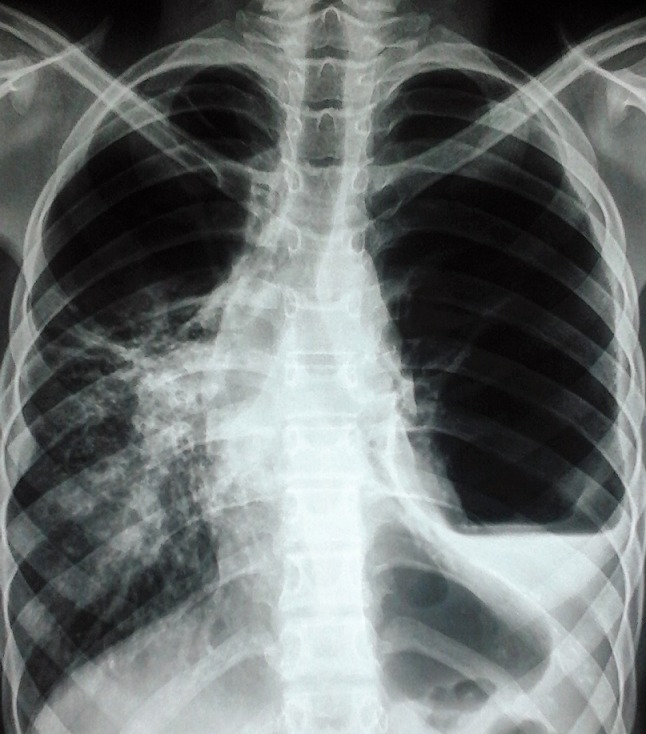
Radiographie thoracique de face aspect du poumon gauche détruit; hémithorax droit: évidement (pneumatocel) du 1/3 supérieur associé à des opacités hétérogènes d’âges différents rétractiles intéressant les 2/3 inférieurs avec déformation du hile et de la courbure de la coupole diaphragmatique
